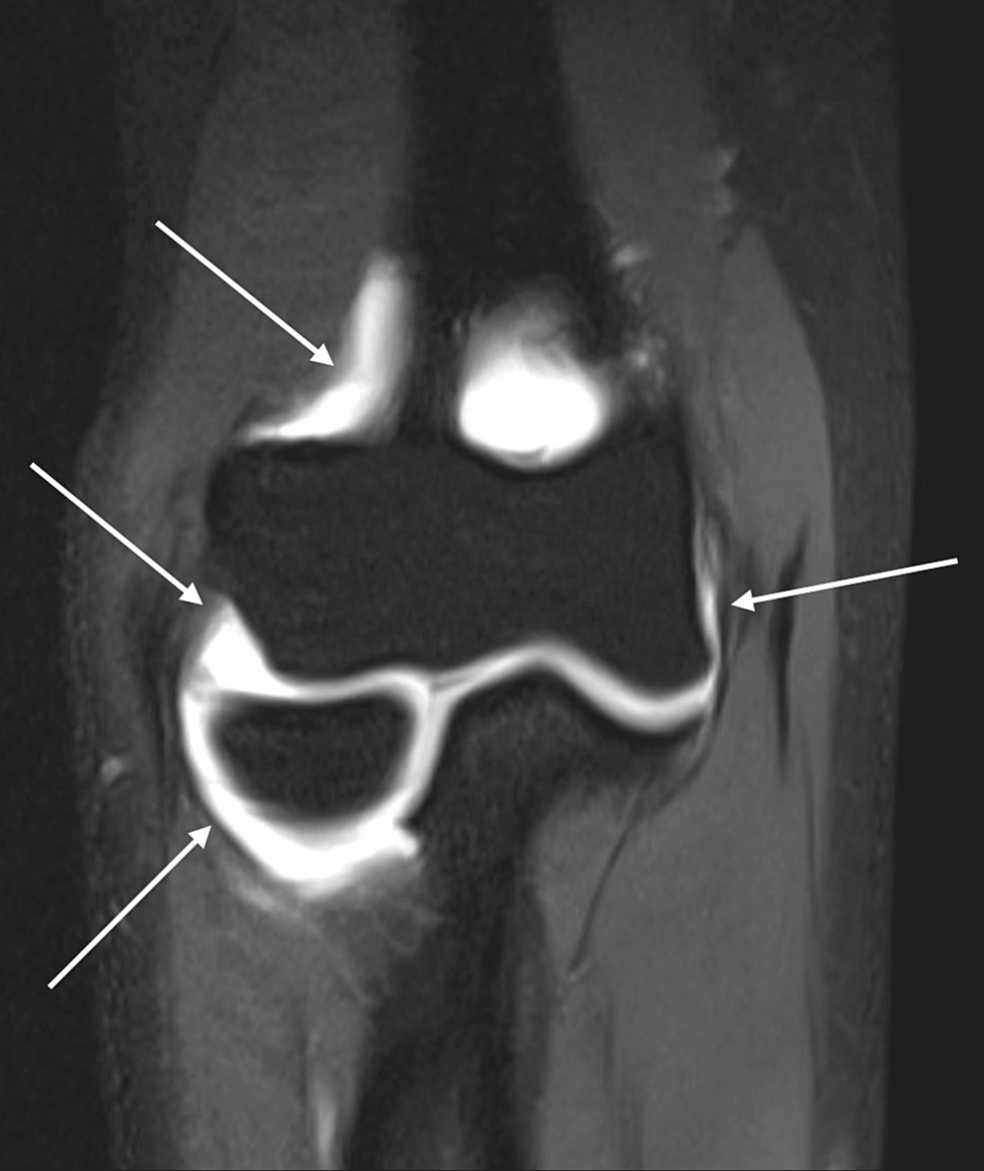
MRI Arthrogram MRI post arthrogram demonstrating adequate distention of the elbow joint capsule (arrows) without extravasation.
